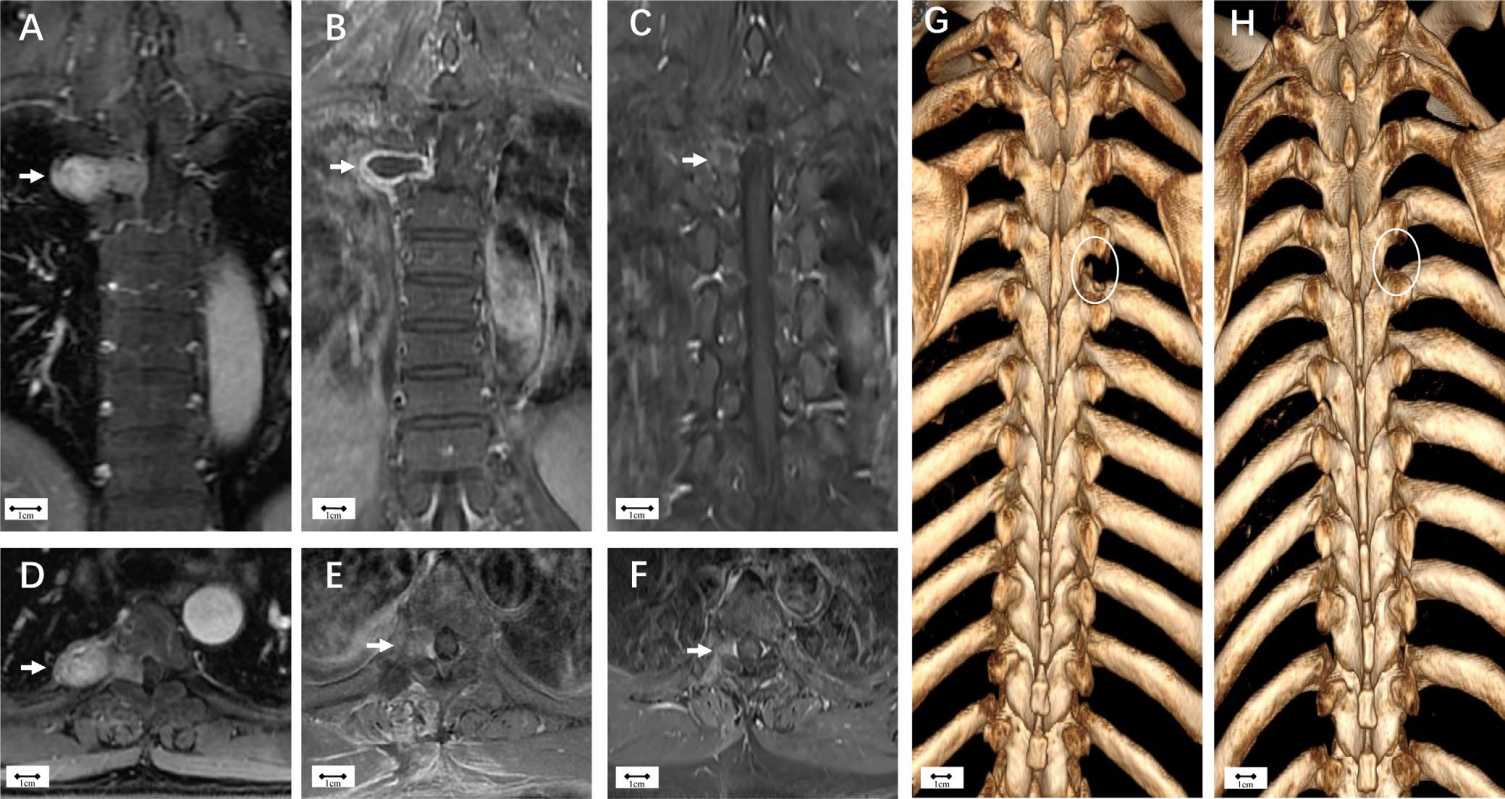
Case II (Eden type III). (A, D) Preoperative enhanced MRI showed a 40 mm × 21 mm × 18 mm mass near the T4/5 spinal column with uneven enhancement. (B, E) Postoperative enhanced MRI showed no tumor residue. (C, F) There was no tumor recurrence at 1‐year follow‐up. (G) Postoperative CT‐VRT‐3D reconstruction indicating destruction of a few ribs, isthmus, and transverse processes while the articular processes remained intact (circle). (H) Follow‐up 1 year later showed partial regeneration of local bones (circle).
